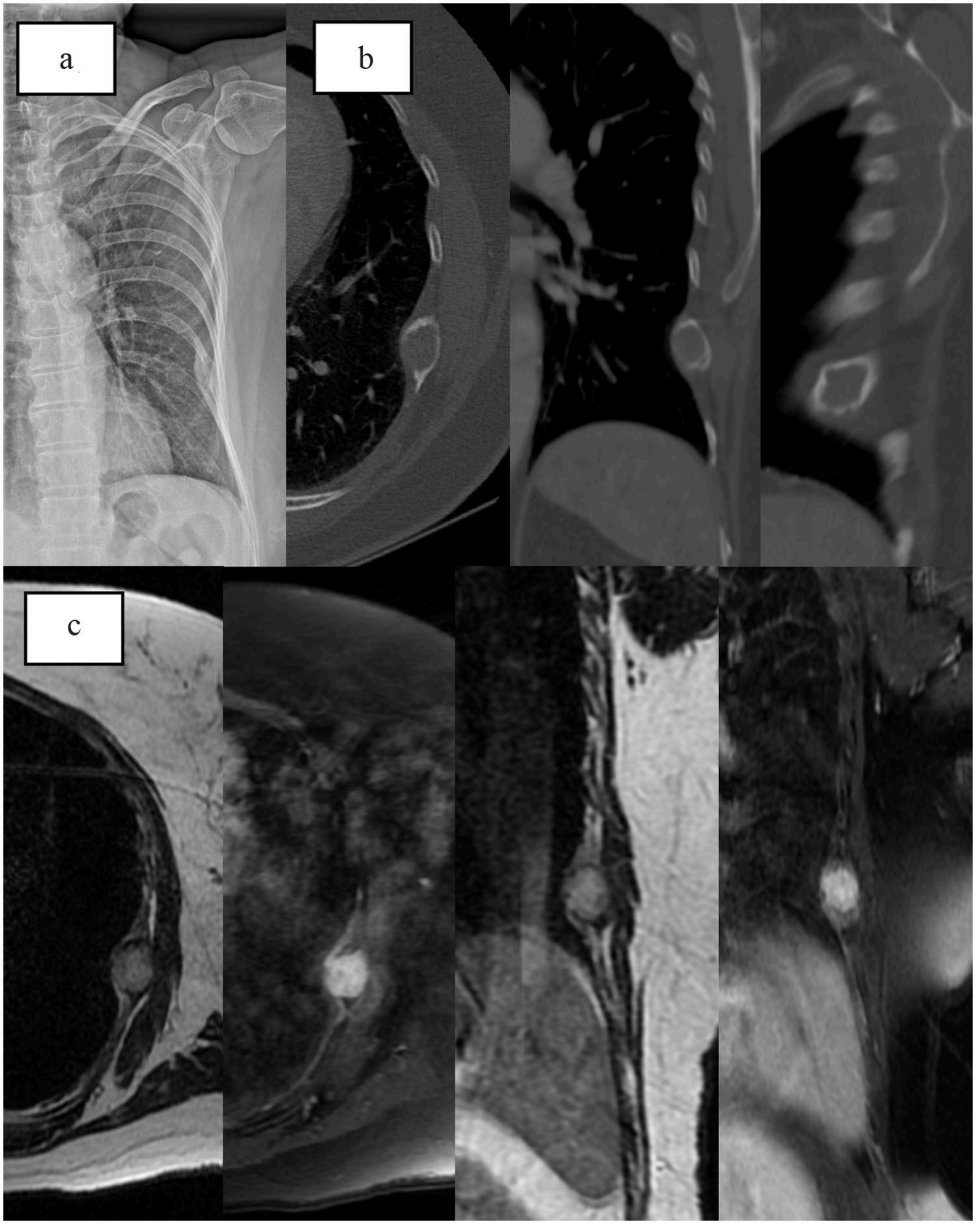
(a) X-ray of the left-sided chest wall in anterior-posterior plane. (b) CT scan of the chest wall in axial, coronal, and sagittal view. (c) MRI of the chest wall in axial (T2, T1 fs with gadolinium) and coronal (T2 and T1 fs with gadolinium) view.
